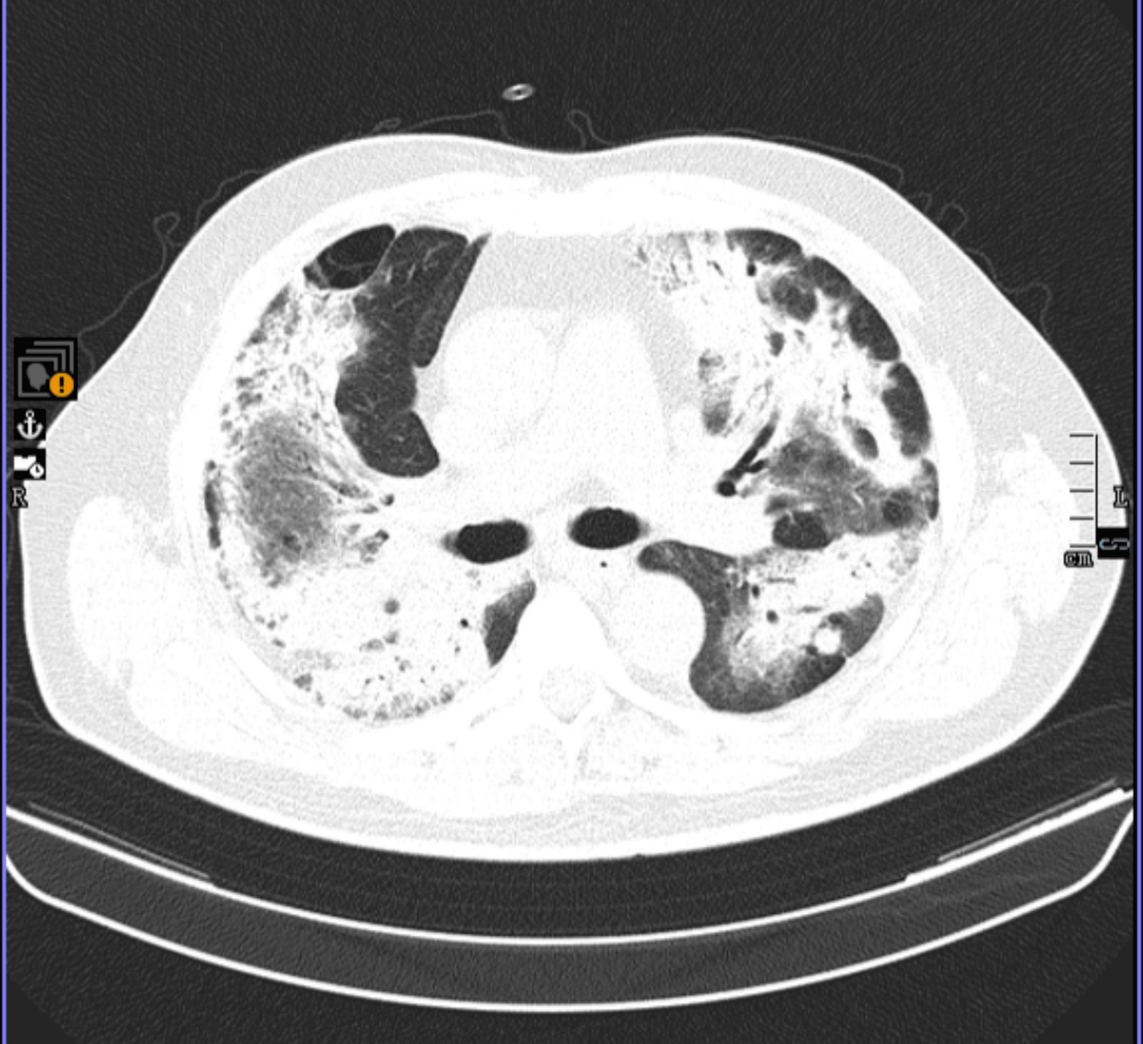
Chest CT (after 14 days of antibiotic therapy) Lung window, axial view. Persistence of bilateral pulmonary infiltrates without significant radiological improvement, reinforcing the differential diagnosis of immunotherapy-related toxicity. CT: computed tomography
